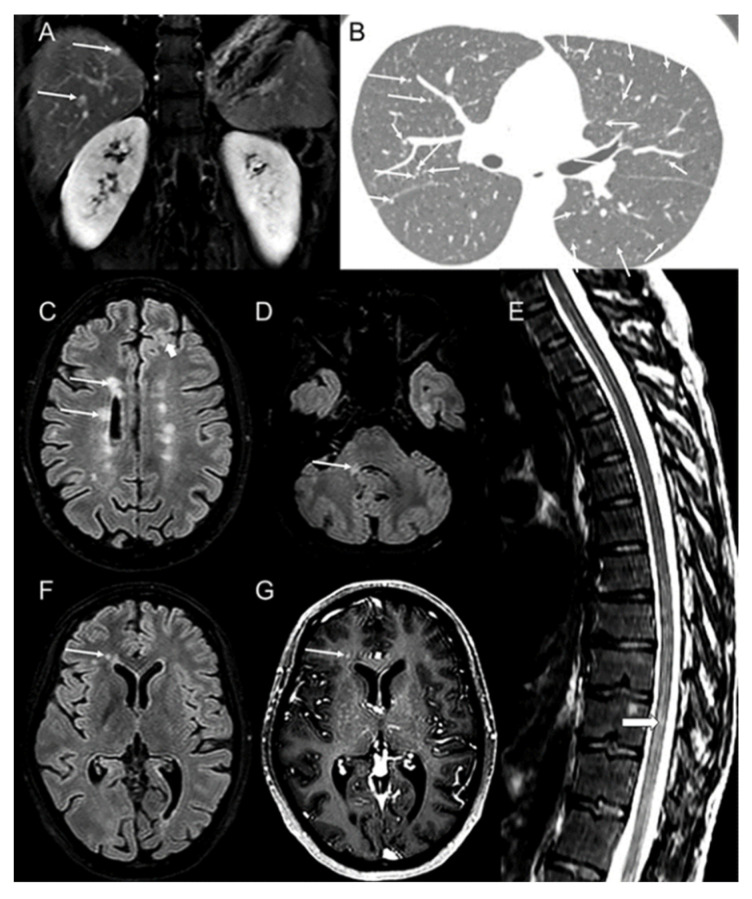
Abdominal MRI reveals hypervascular liver metastases (arrows (A)) from medullary thyroid carcinoma on coronal T1 post-contrast administration image (A). Chest CT (B) shows multiple micronodular lung metastases (arrows (B)). Brain MRI reveals multiple hyperintense and demyelinating lesions on axial FLAIR images (C,D) in the periventricular white matter (arrows (C)), in the subcortical white matter (thick arrow (C)), and in the right middle cerebellar peduncle (arrow (D)). There is also a lesion (thick arrow E)) in the thoracic spinal cord, as seen in the sagittal T2 image (E). One lesion seen on axial FLAIR image (F) near the frontal horn of the right lateral ventricle (arrows (F,G)) enhances axial T1 post-contrast image (G).
